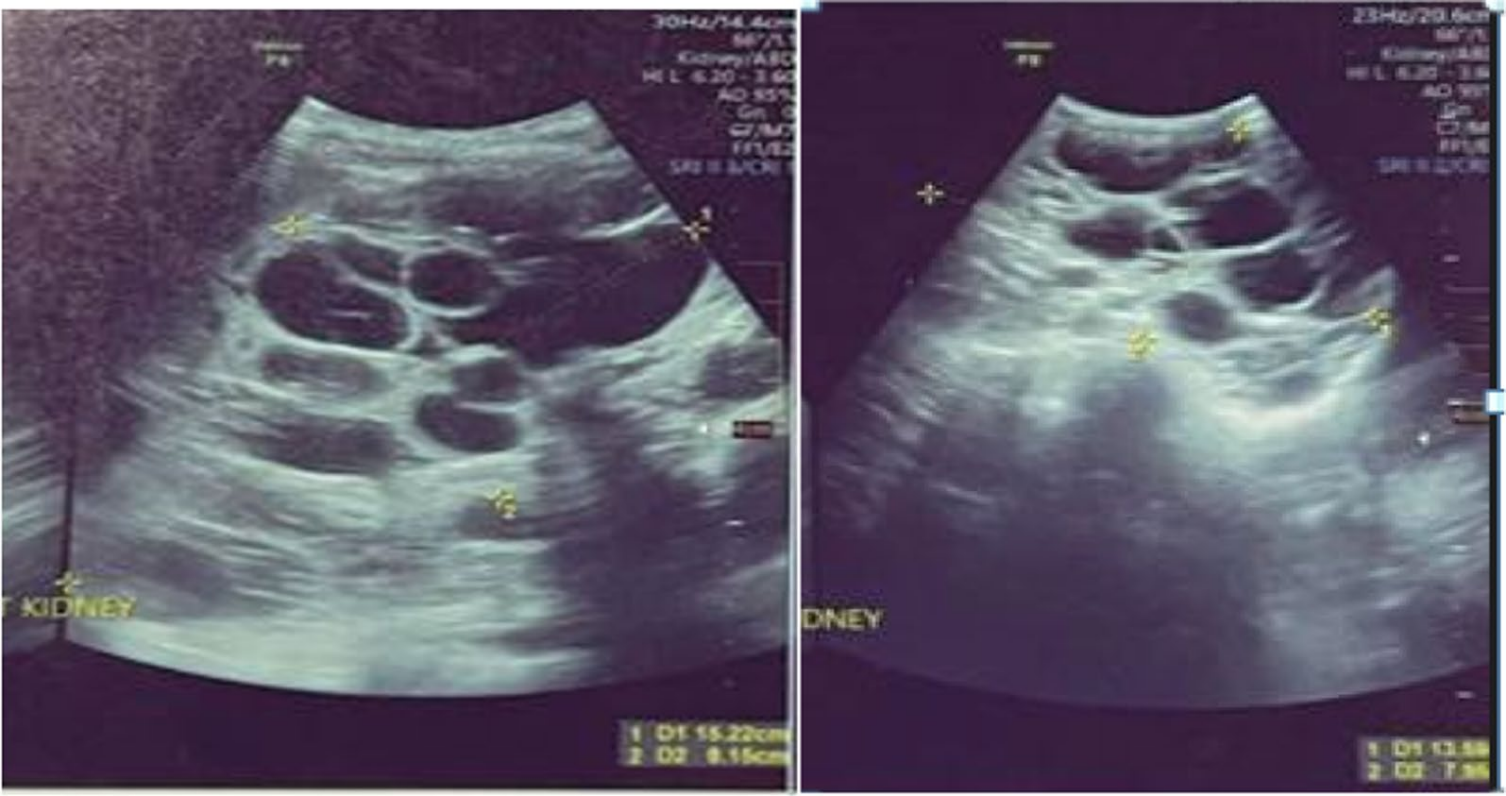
Polycystic kidney on Ultrasound
